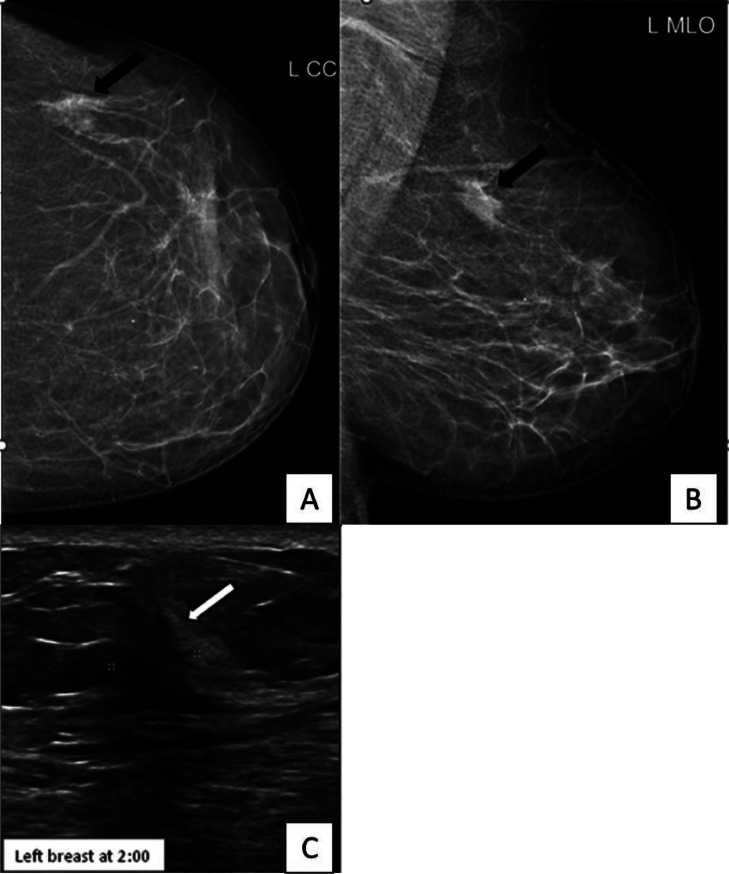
(Imaging of a breast lesion in the left upper outer quadrant). Image (A): Full paddle digital mammography craniocaudal view and image (B): Full paddle digital mediolateral oblique view, both demonstrating an irregular high-density mass at upper outer quadrant (black arrow) classified as BIRADS 5 according to ACR lexicon. Image (C): Axial grey scale ultrasound image of the same lesion shows corresponding irregular hypoechogenic mass at 2:00 measuring 1 cm (white arrow).
